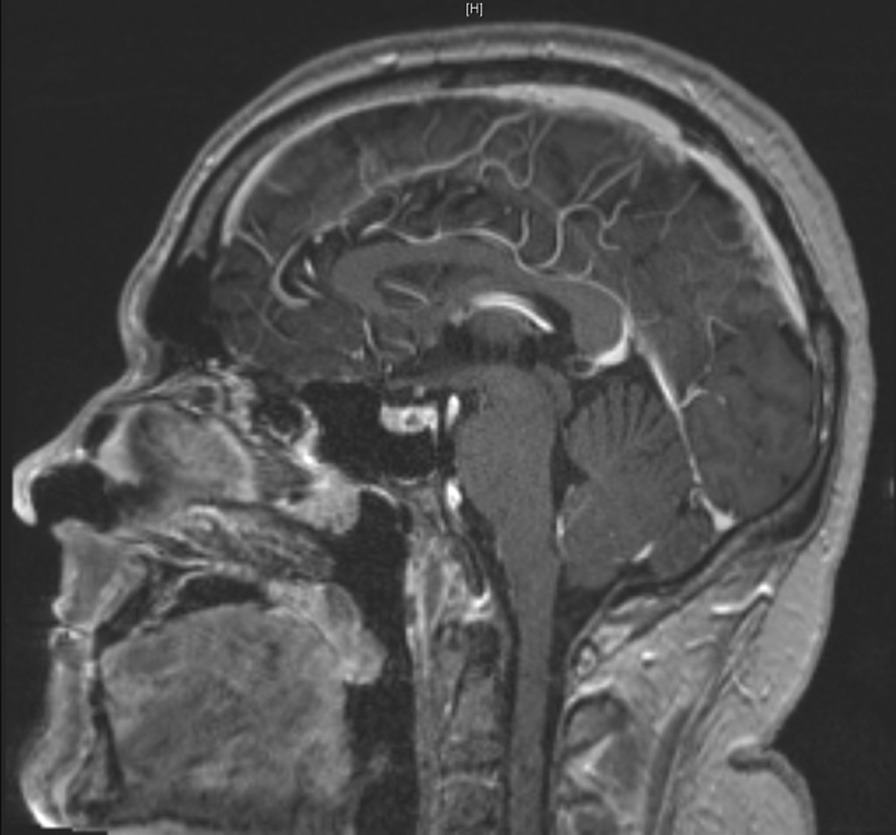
Saggital imaging of the hypophysis on a 3-T T1-weighted MRI image
